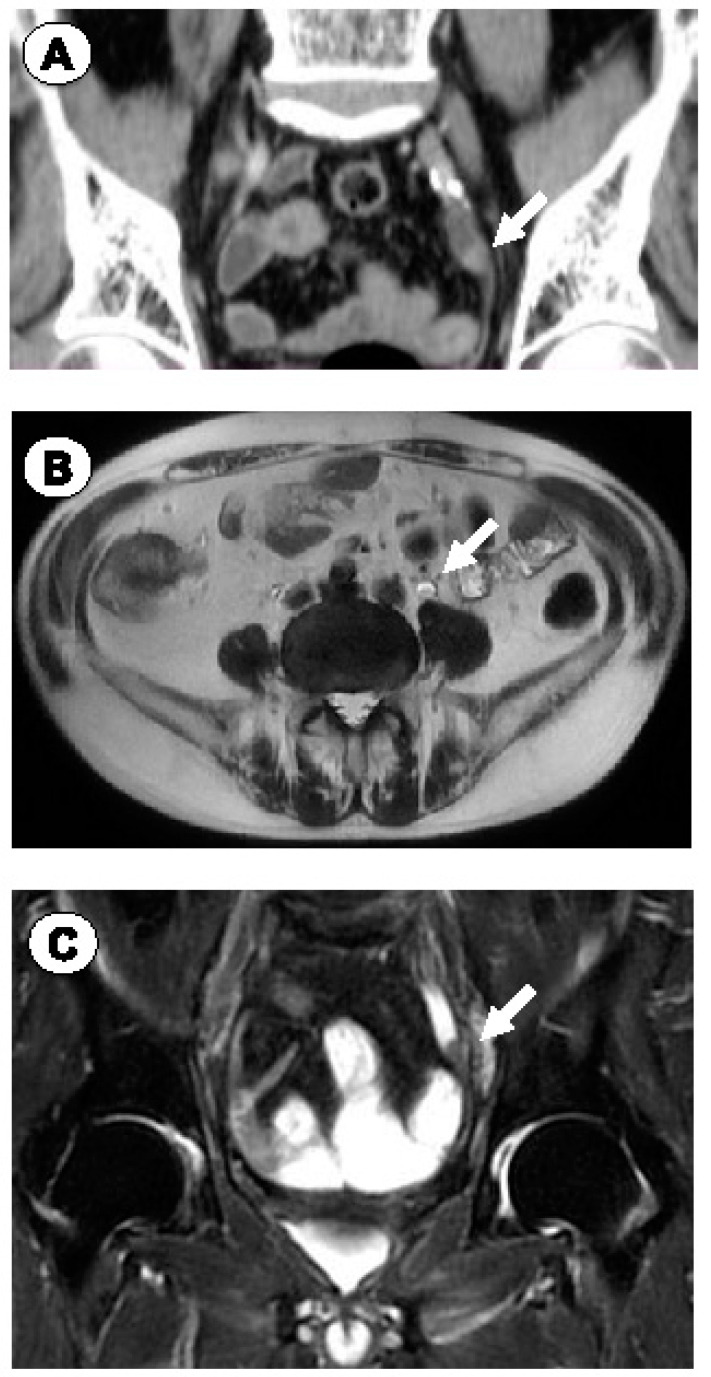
Case 1. Coronal CT (A), and axial (B) and coronal (C) MRI images demonstrating a left ureteral tumor. Arrows indicate the tumor.
